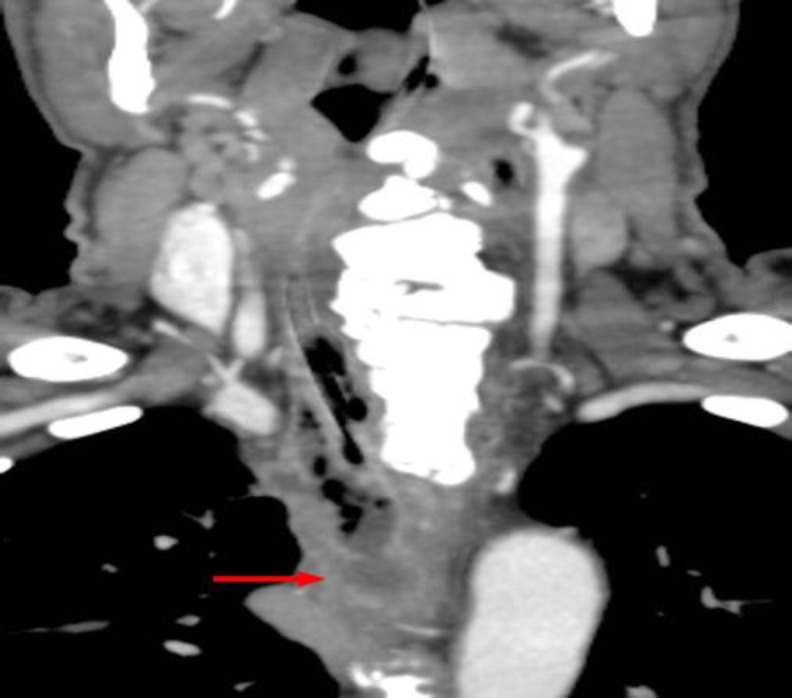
Neck CT scan (coronal scan) showing DISH at the level of C3-T1 and suppurated and fissured diverticulum up to the level of T2 (red arrow)
